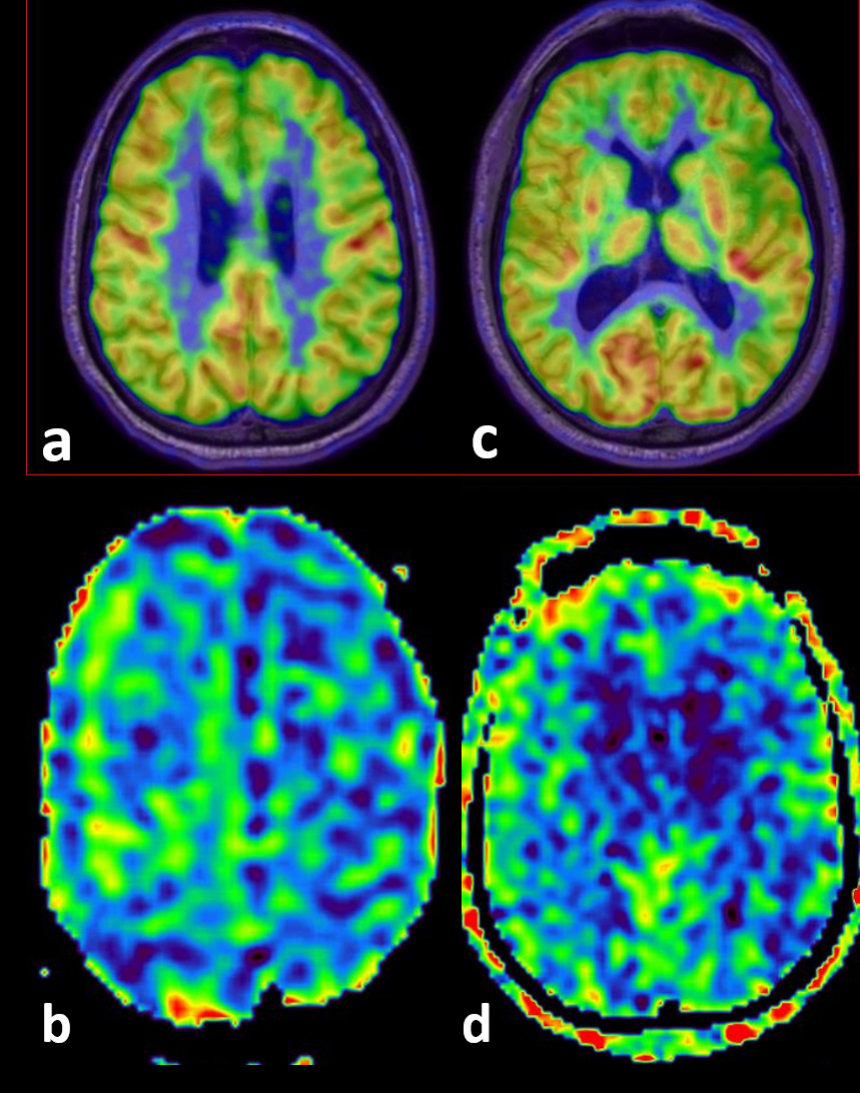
A 53-year-old with an ACE-3 score of 81 shows gross visual concordance between ASL (b, d) and PET (a, b) in areas of significant FDG-PET hypo-metabolism. The corresponding PET and ASL images (a, b) show hypo-perfusion in the left frontal region (approx. Z Score -7.5 on FDG-PET), and images (c, d) show hypo-perfusion in the left parietal region (approx. Z Score -5.5 on FDG-PET)
